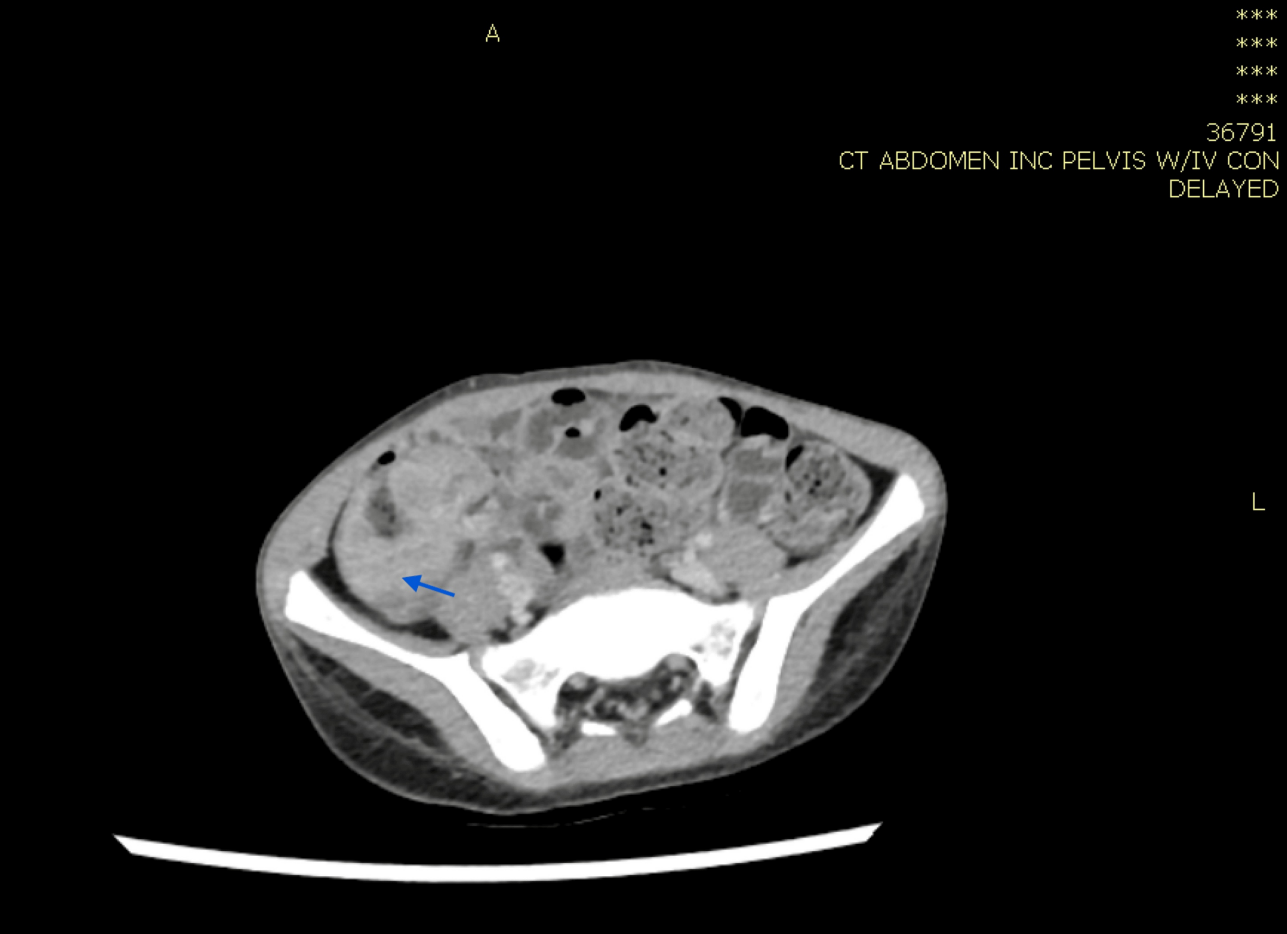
Computed tomography scan of the abdomen with IV contrast showing caecum and proximal ascending colon presenting segments of circumferential moderate wall thickening of approximately 5 cm with significant submucosal oedema and lumen narrowing (blue arrow). Multiple significantly enlarged mesenteric lymph nodes are observed, the largest of which being 1.5 cm in short diameter and 2.3 cm in long diameter, as well as two large focal lesions of the right and left hepatic lobes, associated circumferential thickening and edema of the caecum and proximal ascending colon, and pathologically enlarged necrotic lymph node at the porta hepatis and bowel mesentery. The described findings are suggestive of infection with a high index of suspicion of fungal infection, especially gastrointestinal basidiobolomycosis.
